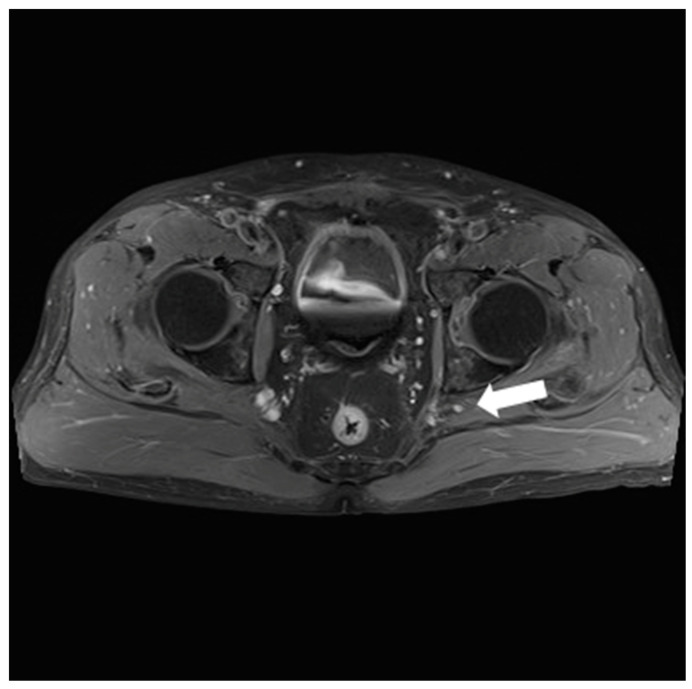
Hip T2-weighted sagittal magnetic resonance imaging (MRI) revealing a cyst measuring 0.5 cm × 0.5 cm next to the sciatic nerve (arrow).
